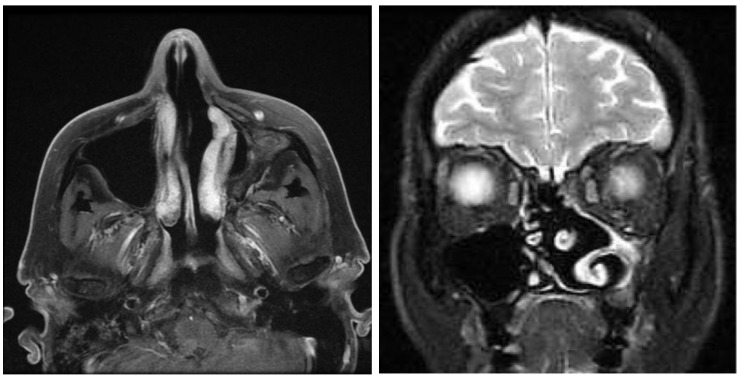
Axial and coronal MRI after prelacrimal approach with a contracted sinus, no signs of recurrence.
